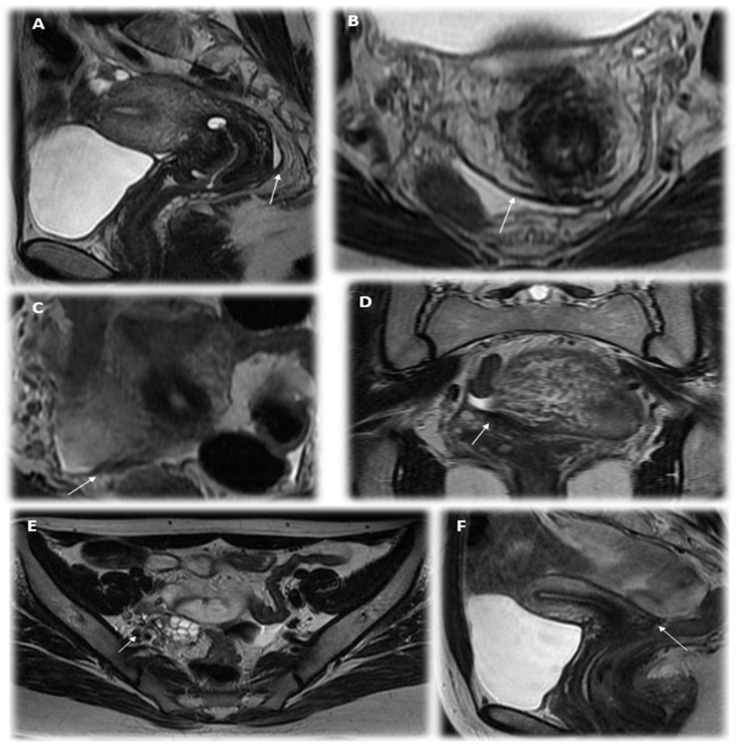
The MRI signs of peritoneal endometriosis in adolescents: (A)—thickening of the peritoneum and partial obliteration of the pouch of Douglas (T2VI Sag); (B)—thickening of the peritoneum of the Douglas space, free fluid and an area of low intensity of the MR signal in the projection of the uterosacral ligament on the left (T2VI Ax); (C)—the thickening of the pelvic peritoneum and the uterosacral ligament on the right (T2VI Ax); (D)—thickening of the peritoneum of the pelvic cavity and the right uterosacral ligament (T2WI Cor); (E)—the asymmetry of the structure and hypointense MR signal in the projection of the sacro-uterine ligament on the right and fixation of the right ovary (T2VI Ax); (F)—a single focus of a hypointense MR signal in the retrocervical space of small sizes (up to 0.3 cm) (T2VI Cor).
